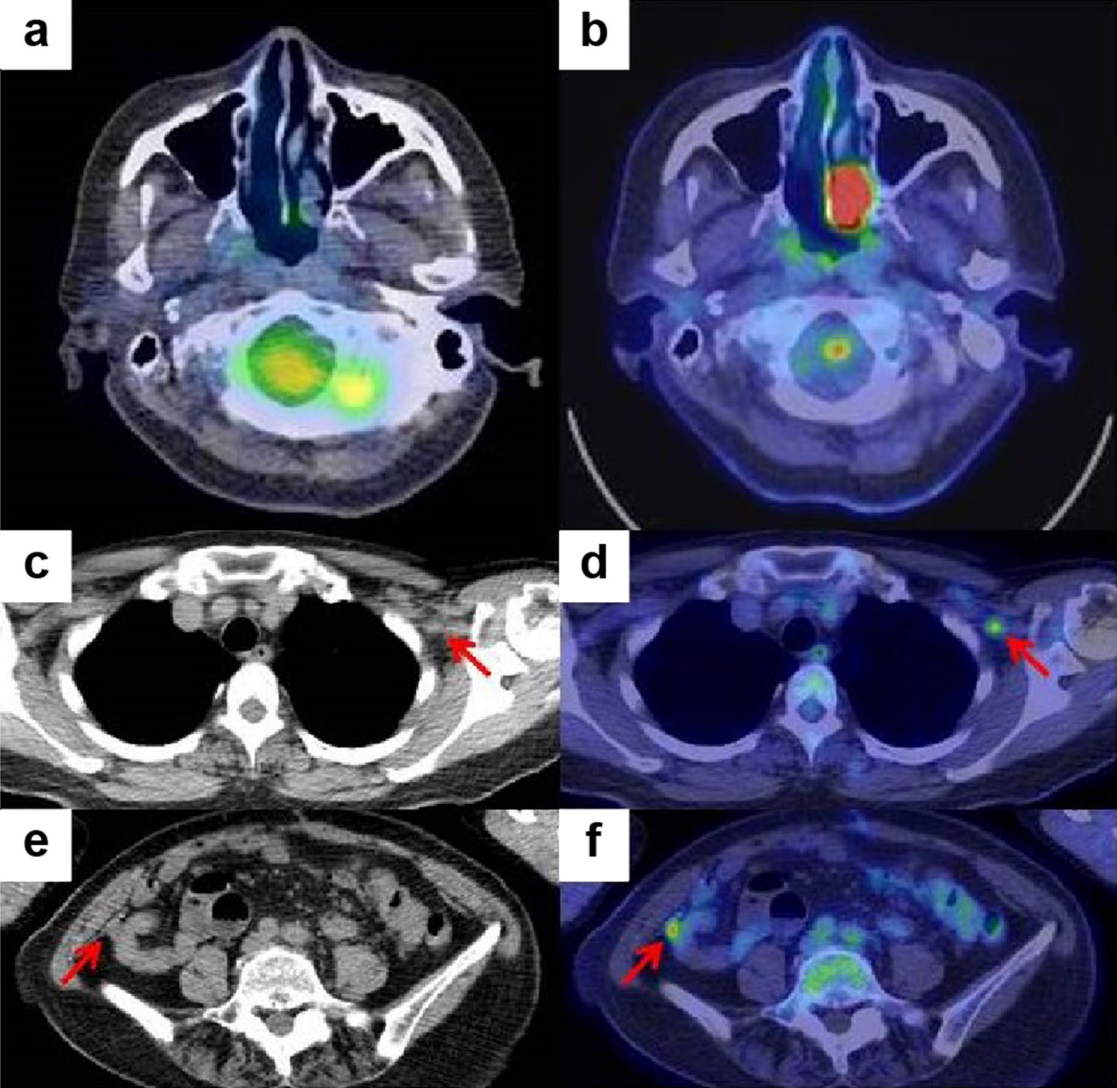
Positron emission tomography–computed tomography image of the nasal cavity before (a) and after surgery (b). Plane computed tomography and positron emission tomography–computed tomography images after surgery revealed left infraclavicular lymph node metastasis (c, d) and a small peritoneal nodule (e, f)
